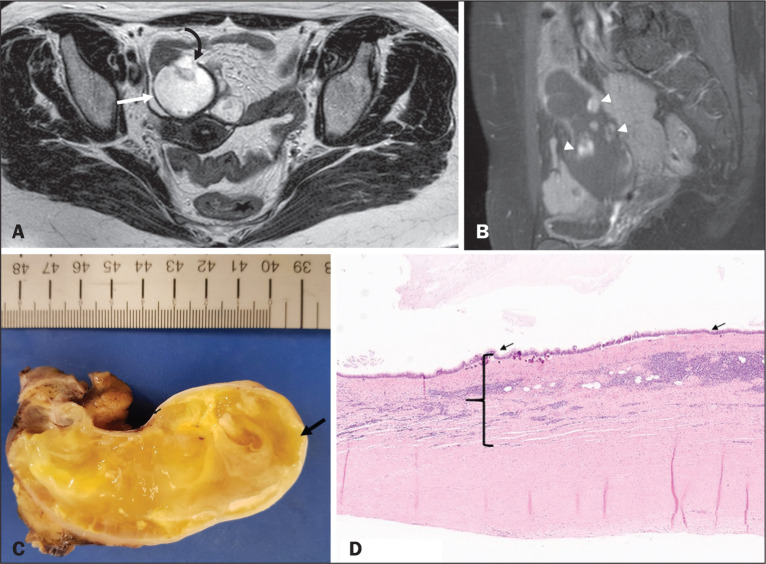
LAMN. Axial T2-weighted MRI sequence (A) and gadolinium
